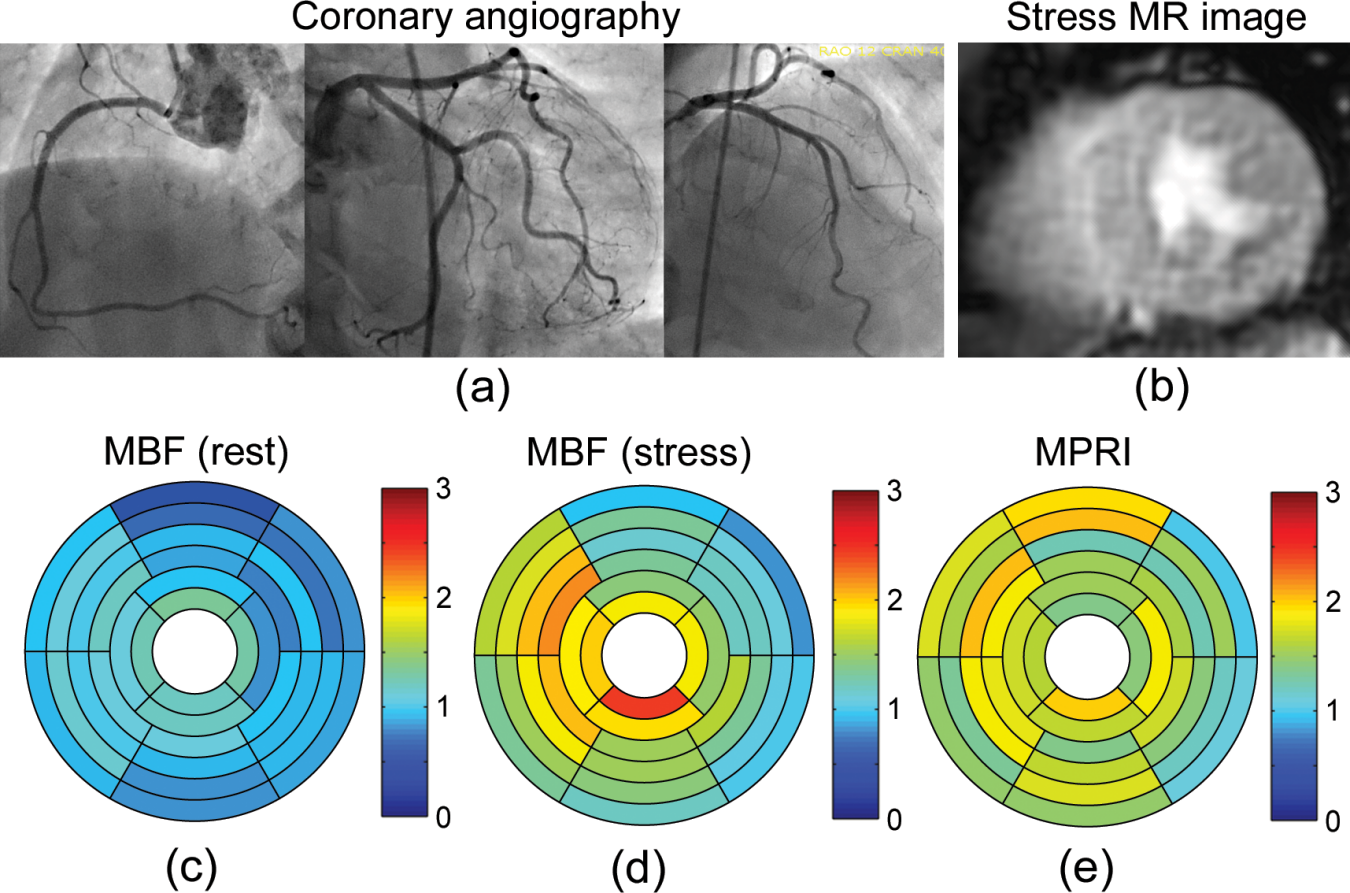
(a) Selective coronary angiography in a representative patient (83-year-old female) with angiographically normal coronary arteries. (b) First-pass perfusion mid-level MR image at stress. Bullseye plots of MBF (ml/g/min) (c) at rest and (d) at stress, and (e) MPRI calculated from the NTH_*f*_ method.
